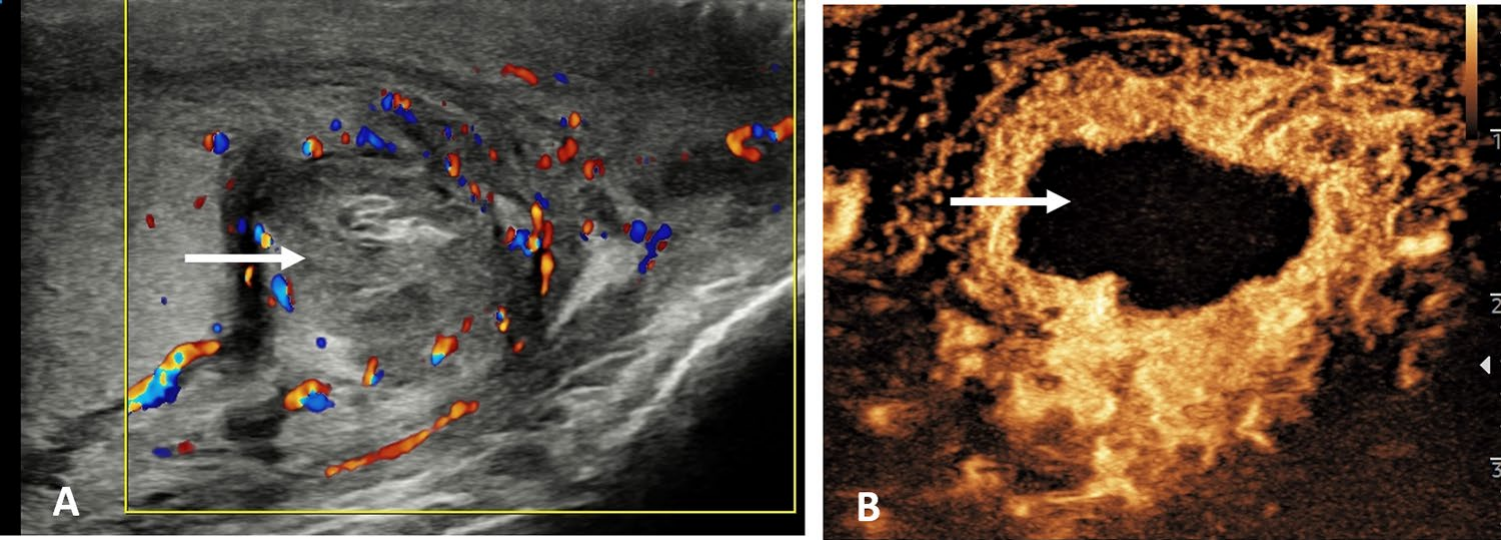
Epididymal abscess in a 56 year old. A Longitudinal view of the right testis demonstrating an echogenic lesion in the epididymal tail (arrow)in epididymitis, with surrounding increase in color Doppler signal. B A contrast enhanced ultrasound examination demonstrates no vascularization in the center of the lesion (arrow) with increased contrast enhancement surrounding the avascular cavity confirming an epididymal abscess
